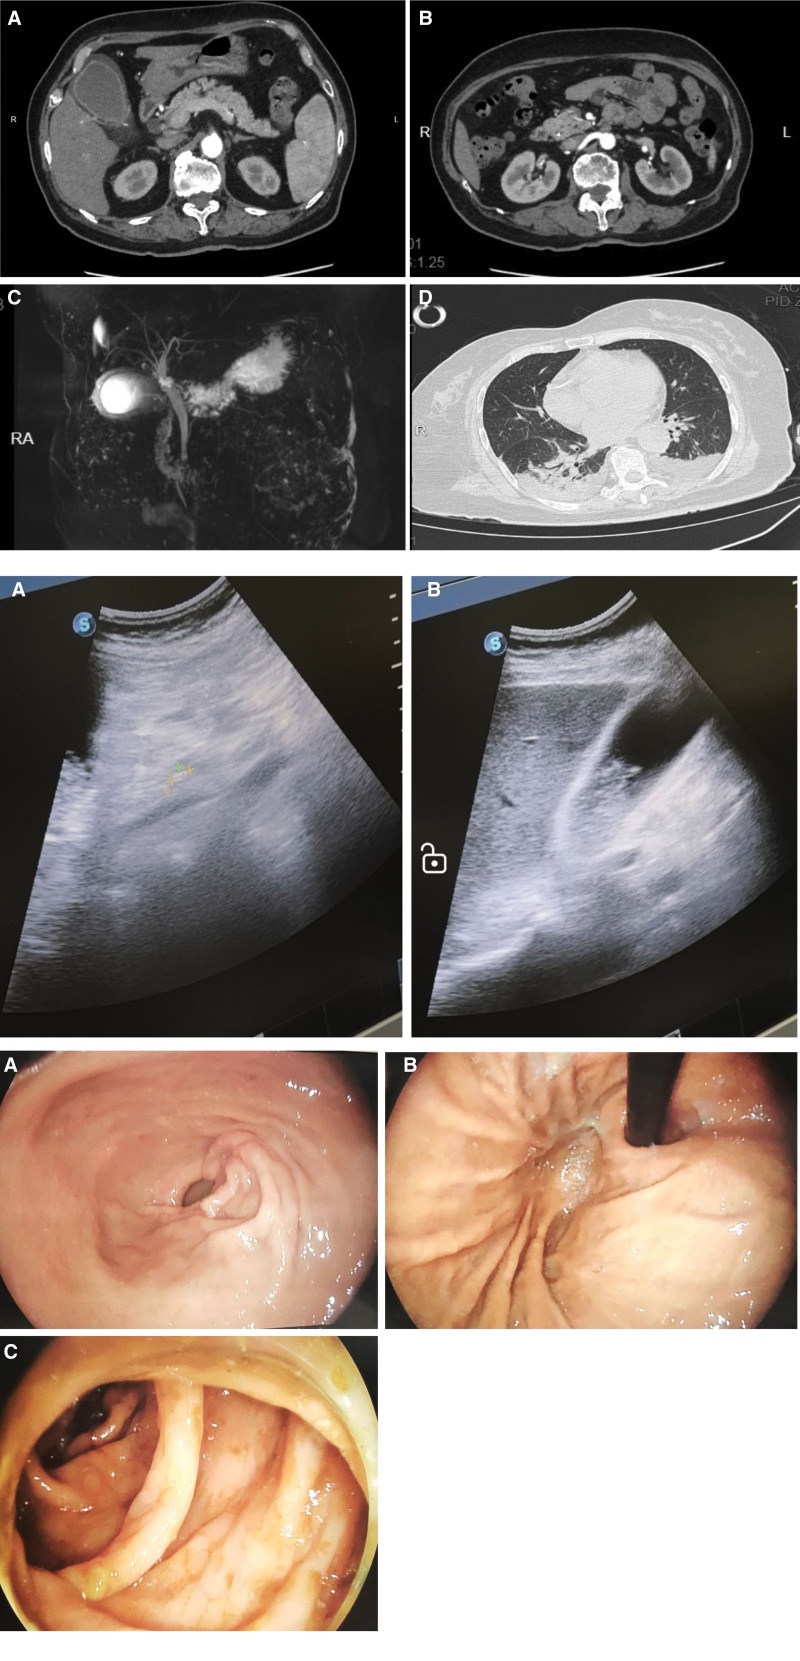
(A. A) Enhanced abdominal CT on March 8, 2024, showed significant cystic edema. (B) Enhanced abdominal CT on March 8, 2024: the common bile duct was dilated and a stone shadow was visible at the lower end. (C) Abdominal magnetic resonance cholangiopancreatography on March 10, 2024: common bile duct dilatation with choledocholithiasis at the lower end of the common bile duct. (D) Lung CT on March 11, 2024: bilateral posterior lower lobe inflammation with pleural effusion. (B. A) Bedside ultrasound on March 11, 2024, revealed a large number of silt-like stones in the gallbladder. (B) Bedside ultrasound on March 11, 2024, which indicated significant bile duct dilatation. (C. A) On March 11, 2024, bedside gastroscope showed no significant bleeding in the antrum. (B) On March 11, 2024, bedside gastroscope showed no significant bleeding in the fundus of the stomach. (C) After bedside colonoscopy on March 11, 2024, no significant bleeding was observed in the colon.
